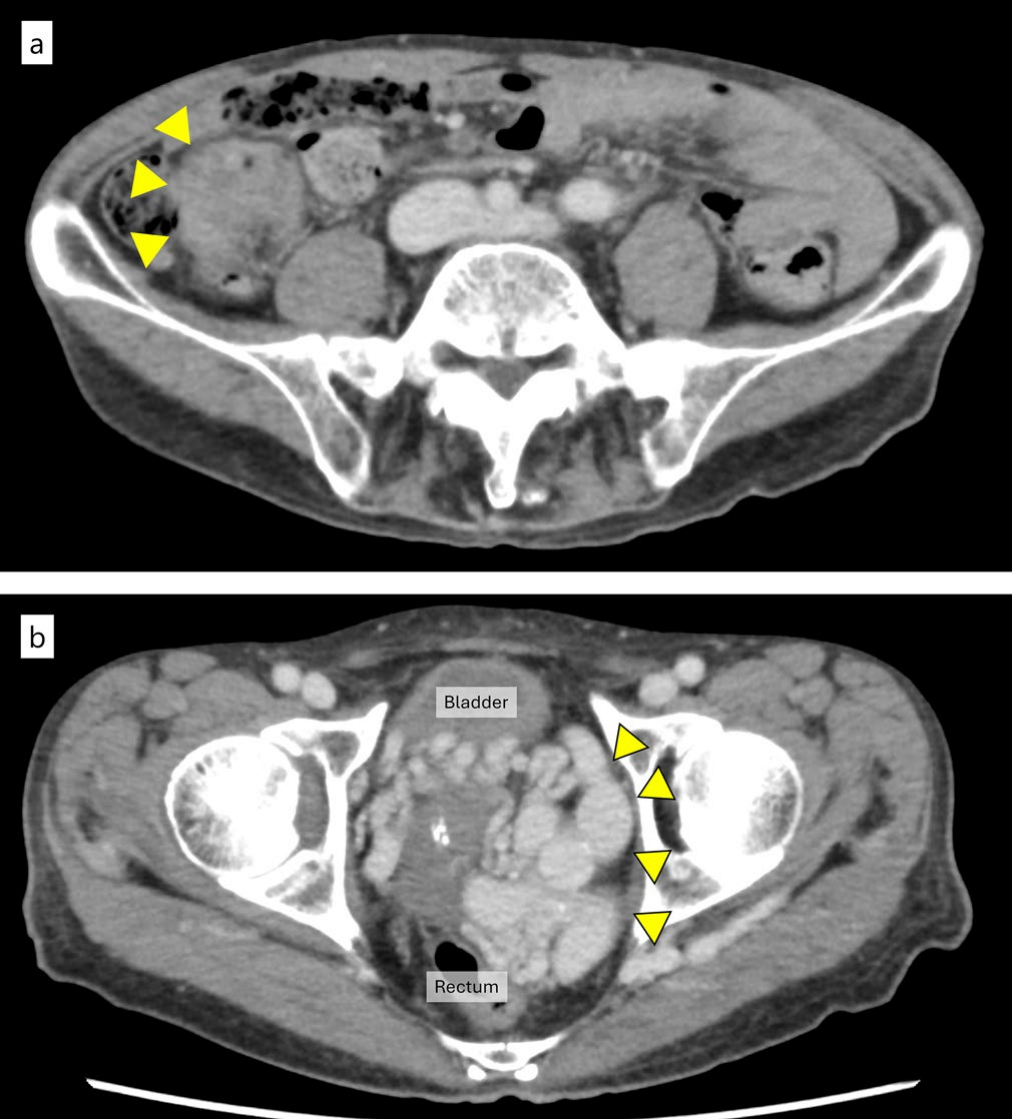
Computed tomography images before surgery. (a) The sigmoid colon was long, and the tumor was located on the right side of its body (yellow arrowheads). There was no obvious lymph node swelling or distant metastasis. (b) Dilated blood vessels are seen on the left side of the pelvis (yellow arrowheads).
